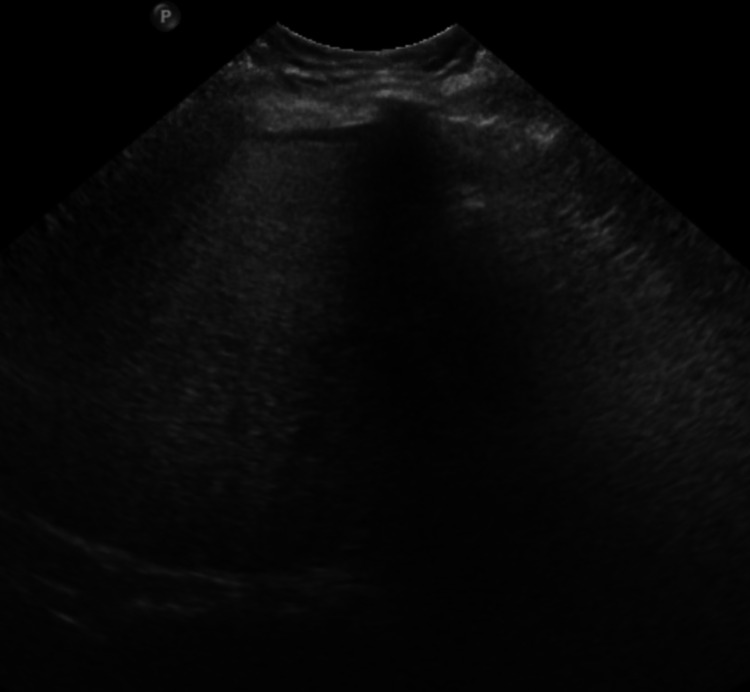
Ultrasound of the liver Abdominal ultrasound showing hepatic steatosis, absence of a gall bladder, and no masses or ascites.
